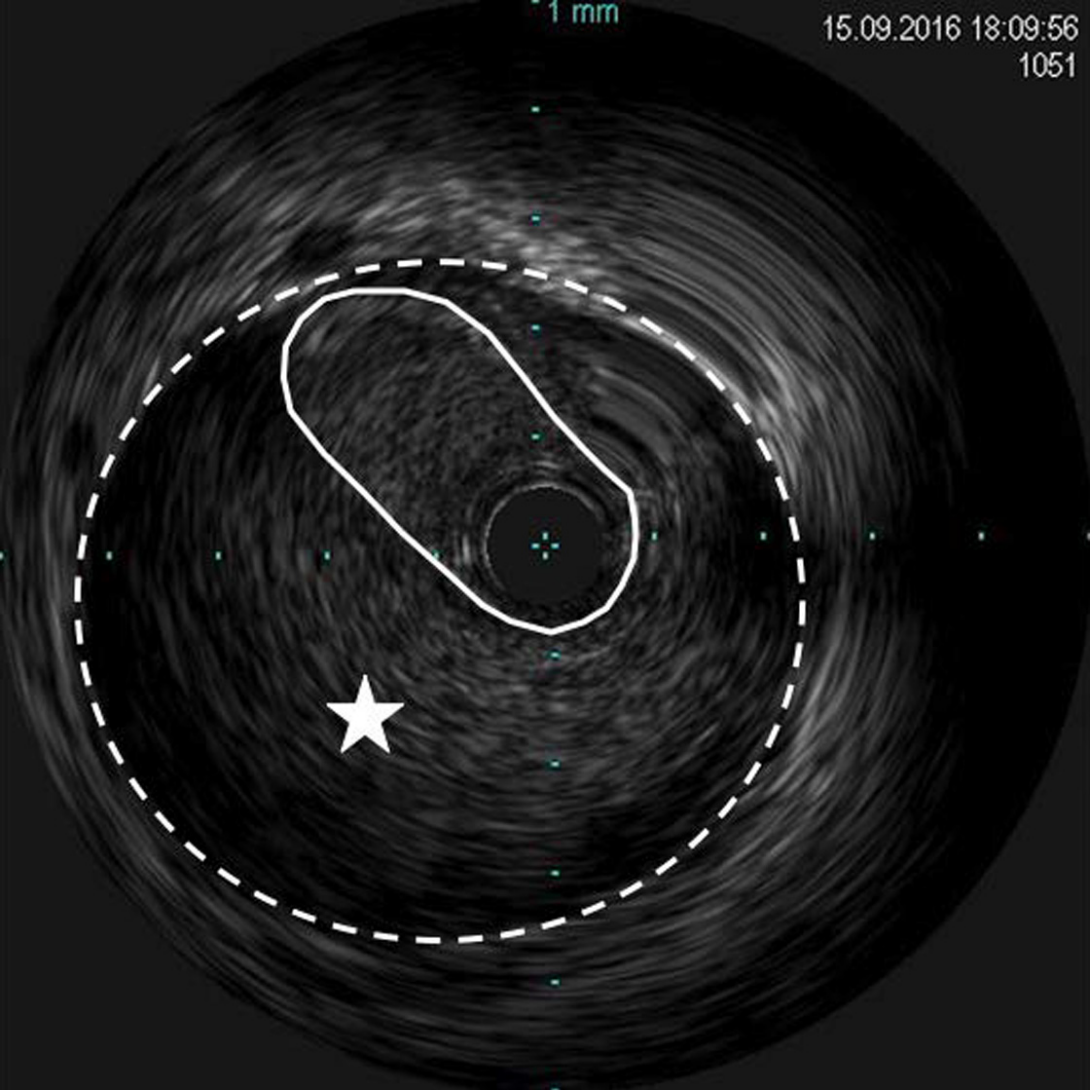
Repeat intravascular ultrasound of the left main coronary artery. The inner region (solid line) denotes the left main lumen. The outer region (dotted line) denotes the adventitia of the left main with evidence of intramural hematoma (star). There is further compromise of the left main lumen.
